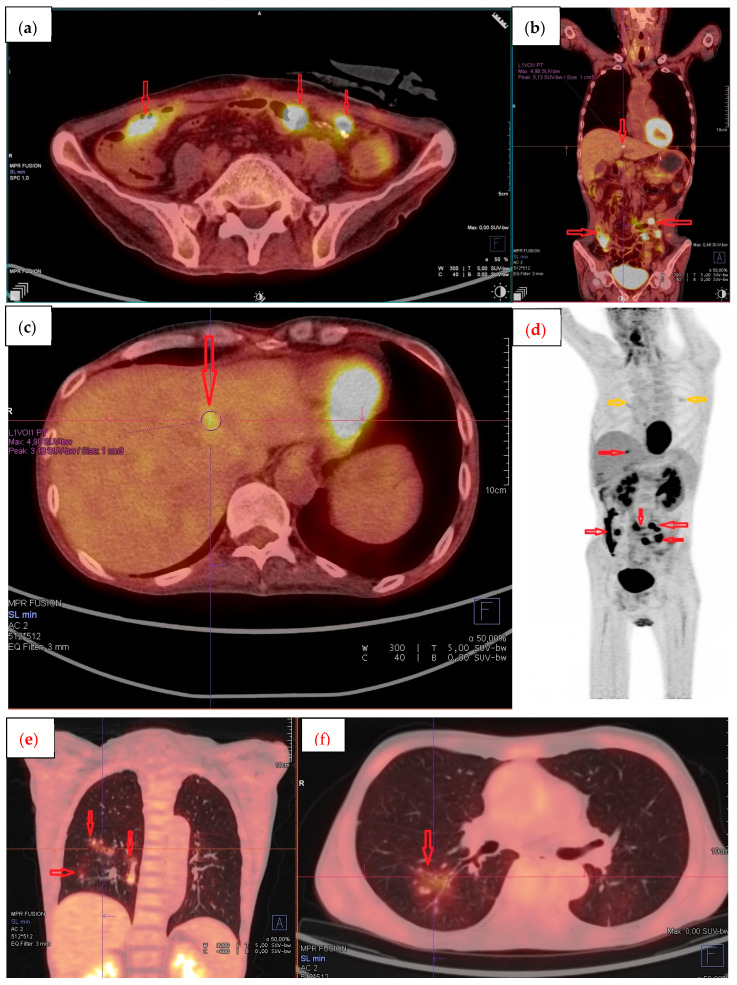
Baseline [^18^F] FDG PET/CT scans before initiation of immunochemotherapy. (a) Axial image showing high tracer uptake in pathological peritoneal and intestinal infiltrations (red arrows). (b) Coronal image with pathological peritoneal and intestinal infiltrations (red arrows). (c) Axial image showing focal tracer uptake in the liver due to lymphoma (red arrow). (d) Coronal image with high tracer uptake in pathological peritoneal and intestinal infiltrations (red arrows); inflammatory changes in the lungs (yellow arrows). (e,f) Axial and coronal images showing diffuse tracer uptake in the lungs due to inflammatory changes (red arrows).
